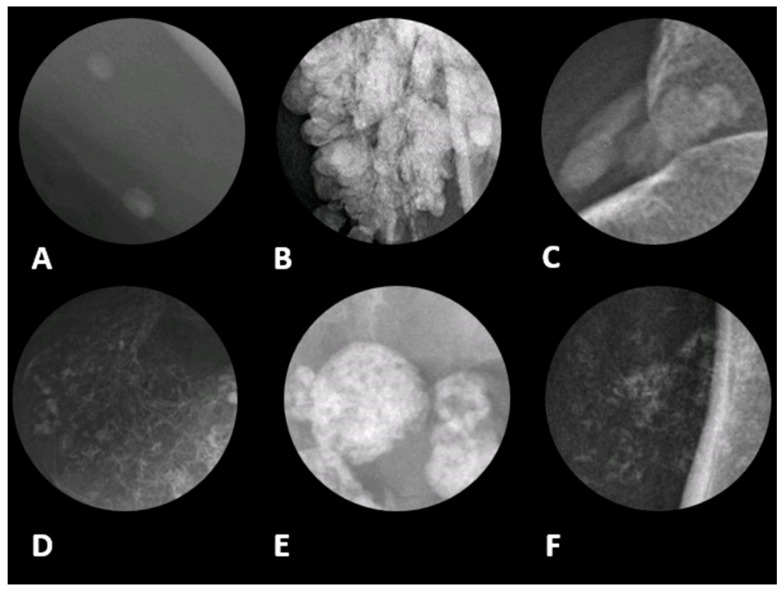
Different radiographic patterns of calcification in soft tissue with associated differential diagnoses in magnified radiographic views. (A) Phleboliths, often appearing as oval or round calcifications and are commonly associated with venous malformations and hemangiomas. It typically indicates a benign etiology. (B) Globular pattern refers to large, lobulated calcifications resembling clusters, seen in tumoral calcinosis, chronic renal failure, and calcinosis universalis. It is likely a benign etiology; however, the exact diagnosis depends on location and clinical history. (C) Amorphous, which is characterized by irregular calcifications without a distinct shape. This pattern is typically seen in dystrophic calcifications resulting from tissue damage, such as calcified tendinitis. (D) Chondroid pattern with rings and arcs calcifications, suggesting a cartilaginous origin. This is characteristic of chondrosarcoma, chondromas, and synovial chondromatosis. (E) Coarse pattern can be found in damaged or necrotic tissues, including certain types of soft tissue sarcomas (F) Stippled or punctate pattern, which appear as multiple small, dot-like calcifications. This pattern can be seen in both benign and malignant tumors. The image was obtained from the University of Washington Medical Imaging database with the required permissions.
